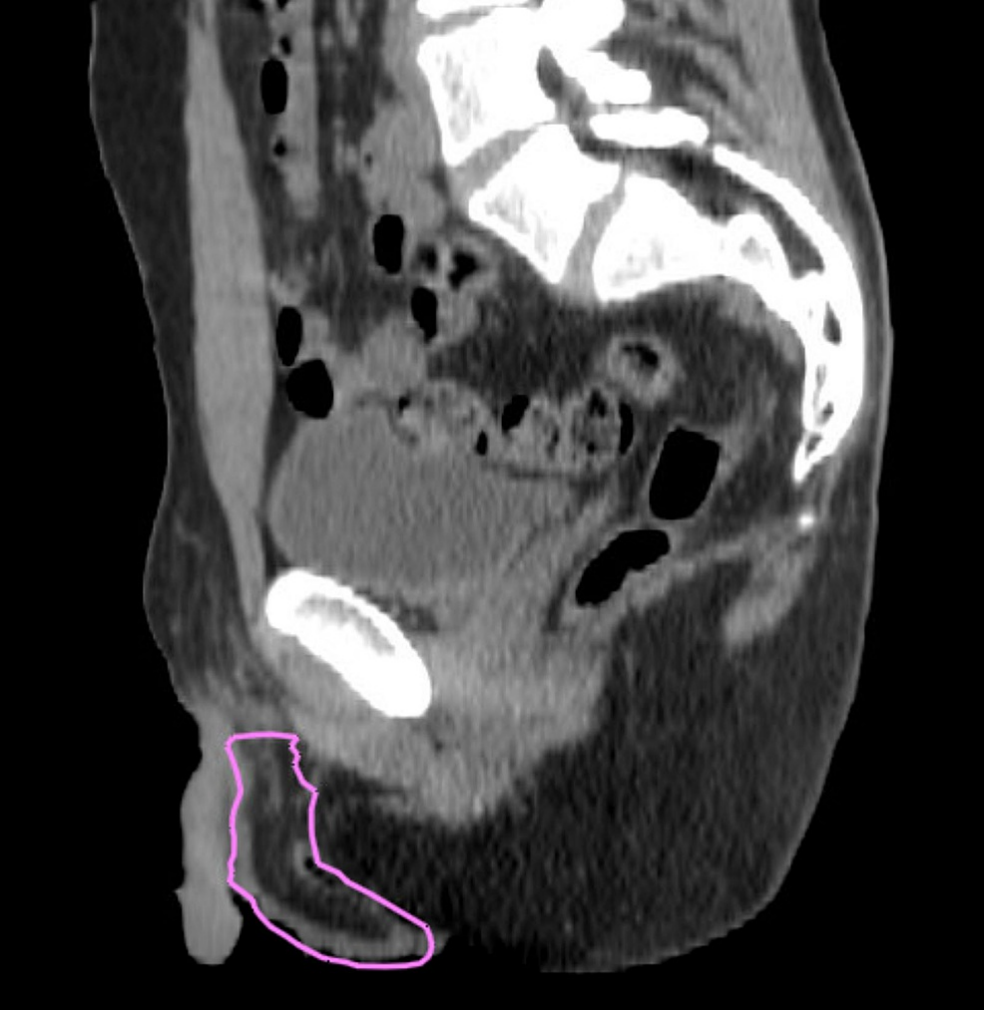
Radiation treatment volumes CT sagittal view of radiation target volumes treated with clinical setup. Pink line represents the clinical target volume. CT: computed tomography
